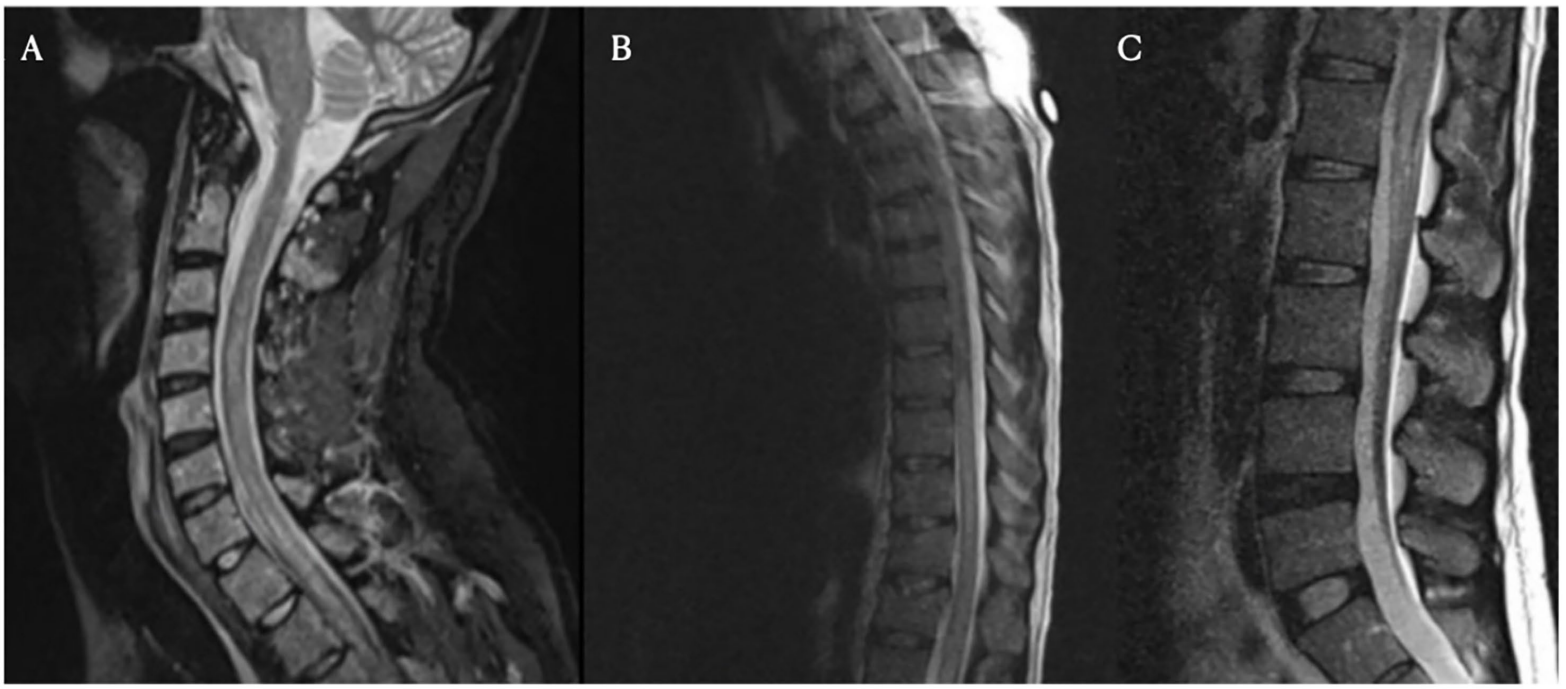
Spinal magnetic resonance imaging on day 10 post-treatment. Cervical (Panel A), thoracic (Panel B), and lumbar (Panel C) spine MRI images obtained on day 10. These images show longitudinally extensive T2 hyperintensity within the spinal cord, most prominent from C7 to T4 and from T8 to the conus medullaris. No significant spinal canal or neural foraminal stenosis is noted at any level.
